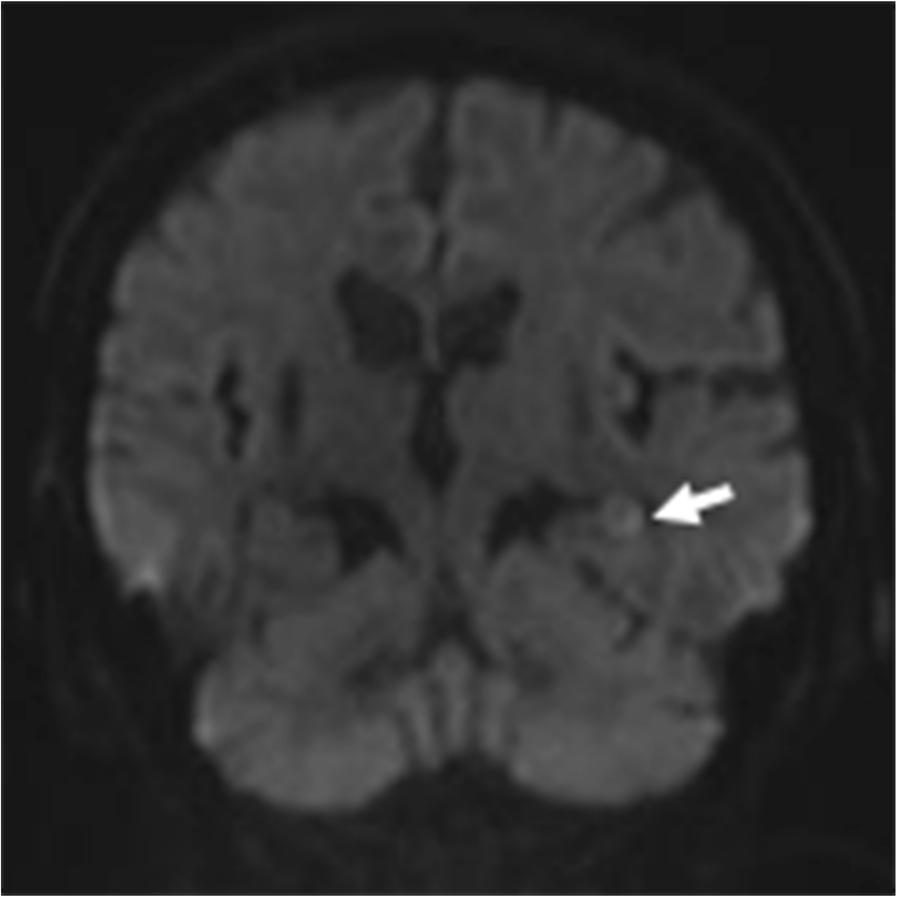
(Coronal) Diffusion-weighted images (DWIs). DWI magnetic resonance imaging scans showing a high-intensity lesion in the dorsal part of the left hippocampal body (arrow).
